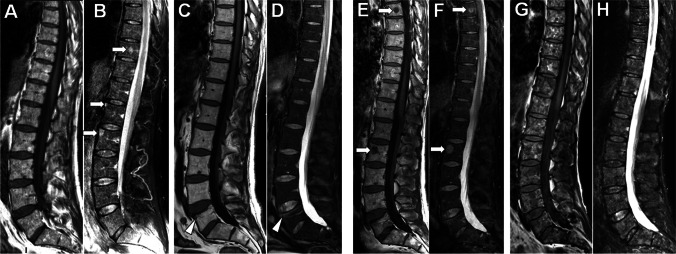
53 year-old man with newly diagnosed MM (IgG A kappa, stage III, ISS 1): MRI findings at baseline, post ASCT, and at relapse. A, B Baseline sagittal T1- (A) and STIR-weighted (B) MR images of the whole spine show diffuse heterogeneous and relatively low signal intensity of the bone marrow on T1, with heterogeneous signal and high signal intensity foci best seen on STIR (arrows in B). C, D Corresponding MR images obtained 12-m later after high dose cytotoxic treatment and ASCT (complete biological response) show complete return to normal signal intensity of the bone marrow. Note the appearance of a new “benign looking” vertebral fracture involving the L5 vertebral body (arrowhead in C and D). E, F Follow-up sagittal T1- (E) and STIR-weighted (F) MR images obtained 6-m later during maintenance therapy show reappearance of several foci of low/high signal intensity (arrows in E, F). G, H Follow-up sagittal T1- (G) and STIR-weighted (H) MR images obtained 12-m later after second line treatment show disease progression with severe increase in marrow infiltration and focal lesions
